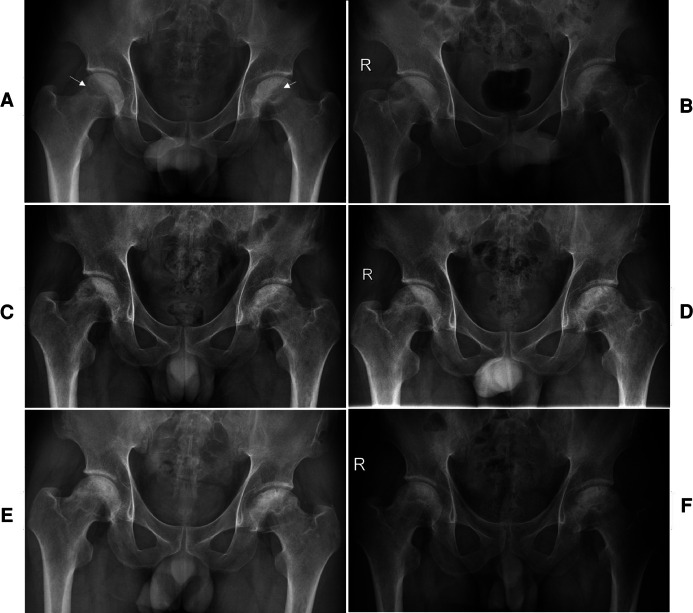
The preoperative x-ray shows cystic and sclerotic changes in the bilateral femoral heads (white arrow) (A). A series of postoperative x-ray (B, immediate), (C, 3 months), (D, 6 months), (E, 12 months), and (F, 24 months) show a gradual healing of the necrotic area at the bilateral femoral heads.
